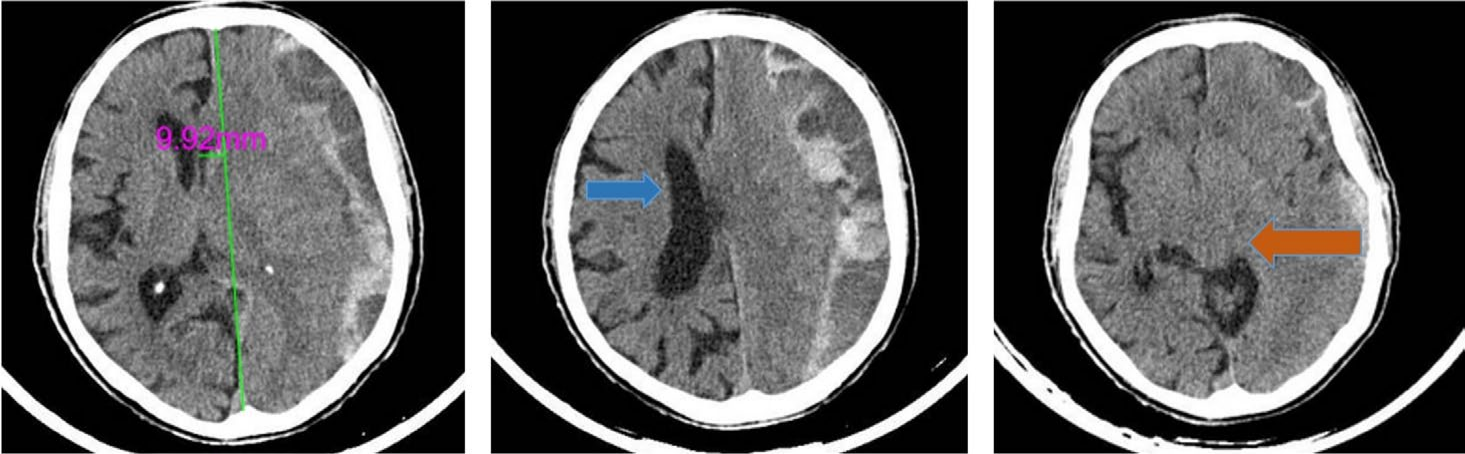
Preoperative Brain CT scan. Left image: The midline shift is about 10 mm. Middle image: The right lateral ventricle is visible (blue arrow), but due to hematoma, the left one is not seen, and the sulci on the left side are effaced. Right image: The red arrow shows that the left basal cisterns are compressed.
